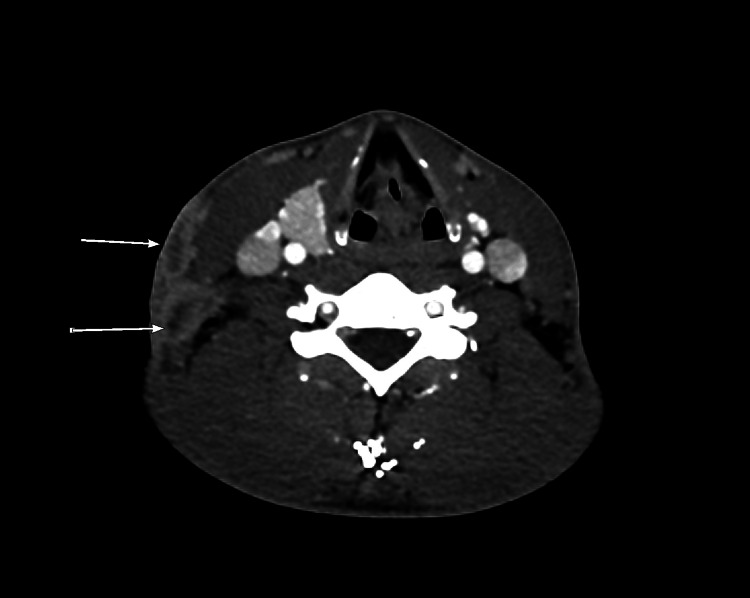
CT scan neck (axial) after about five months of treatment Interval development of left lower cervical, left supraclavicular, and left paratracheal necrotic lymph nodes extending down into the mediastinum.
